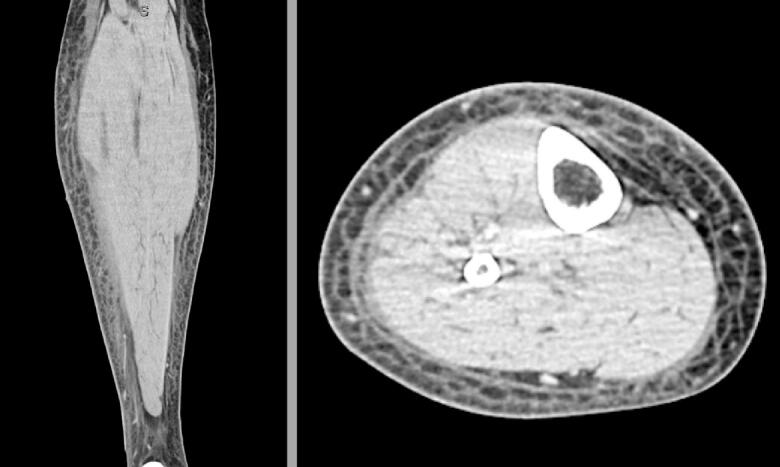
Coronal and axial view of CT scan of patients right lower extremity. First read at outside hospital was remarkable for subcutaneous edema consistent with cellulitis. Second opinion read at second hospital concluded cellulitis with no evidence of abscess or bone/joint abnormality.
